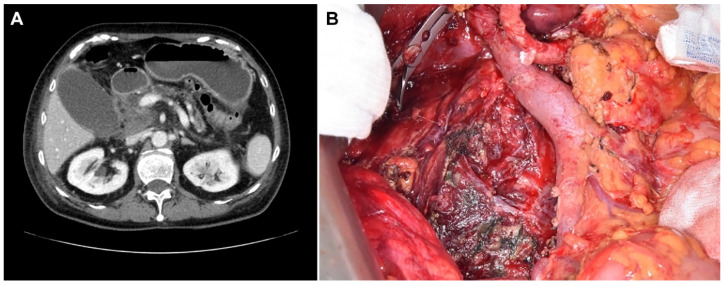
Preoperative image and operative findings of a PAP patient with a successful pancreatoduodenectomy. (A) Preoperative computed tomography (CT) image showing post-ERCP pancreatitis. (B) Operation findings of the patient after pancreatoduodenectomy showing debris of necrotic tissues.
